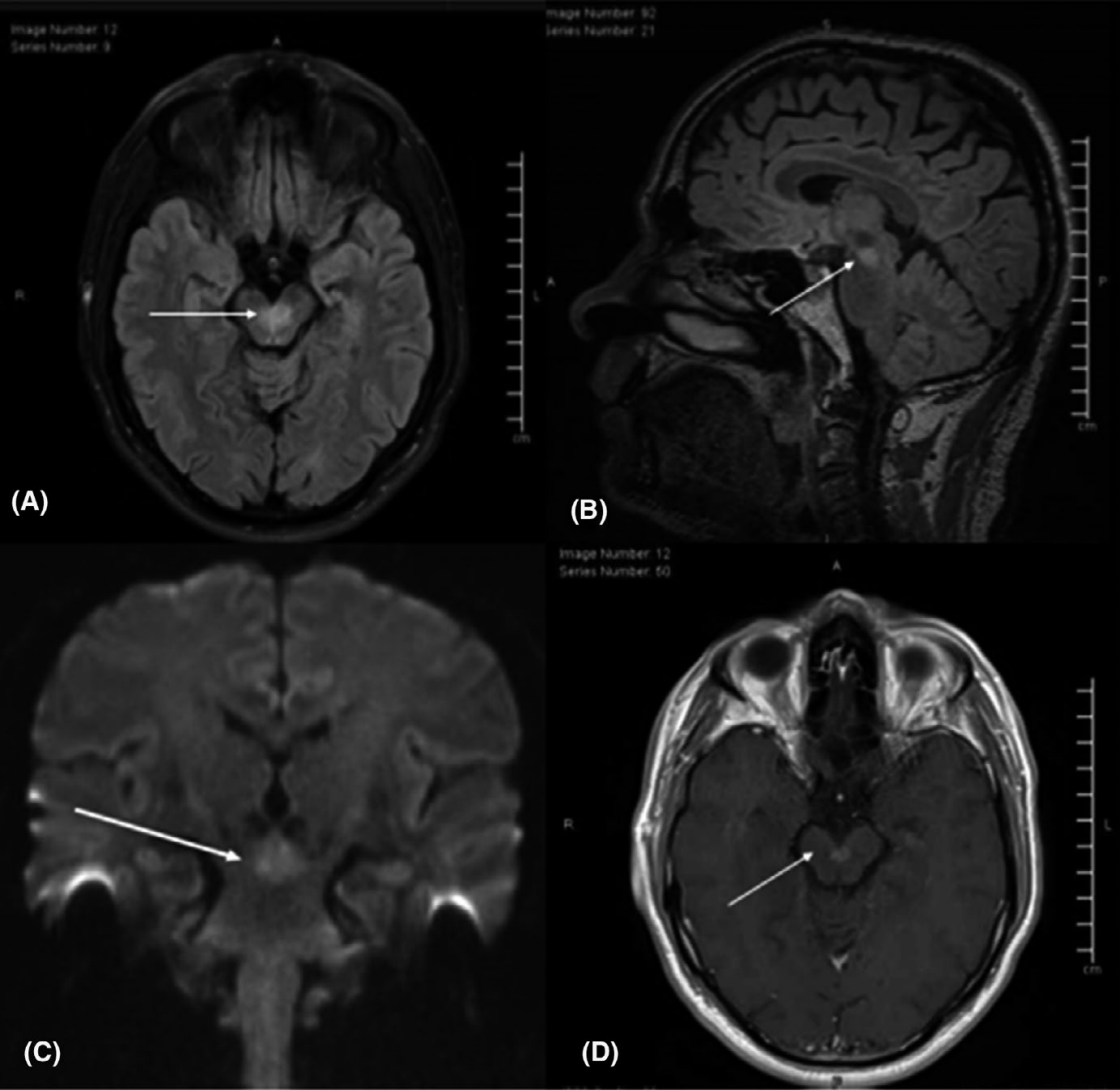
Magnetic resonance imaging (MRI) brain, (A) axial FLAIR‐T2 (fluid attenuated inversion recovery), coronal diffusion‐weighted series long b value (B), and apparent diffusion coefficient (ADC) (C), and axial T1‐weighted (T1WI) post I.V. Gadolinium‐based contrast (Gd), demonstrates a midbrain midline lesion centered at the brachium conjunctivum just posterior to the interpeduncular cistern appears, with high T2‐FLAIR signal intensity, facilitated diffusion, and homogenous post‐contrast enhancement, with minimal perilesional edema. It measures about 5 × 8 × 6 mm in anteroposterior, transverse, and craniocaudal dimensions, respectively (arrow in A, B, C, and D). The appearances raised possibility of biological low‐activity neoplastic process such as lymphoma, metastasis or glial tumor, solitary active demyelinating lesion or neuro‐inflammatory such as neurosarcoid or Behcet's disease
